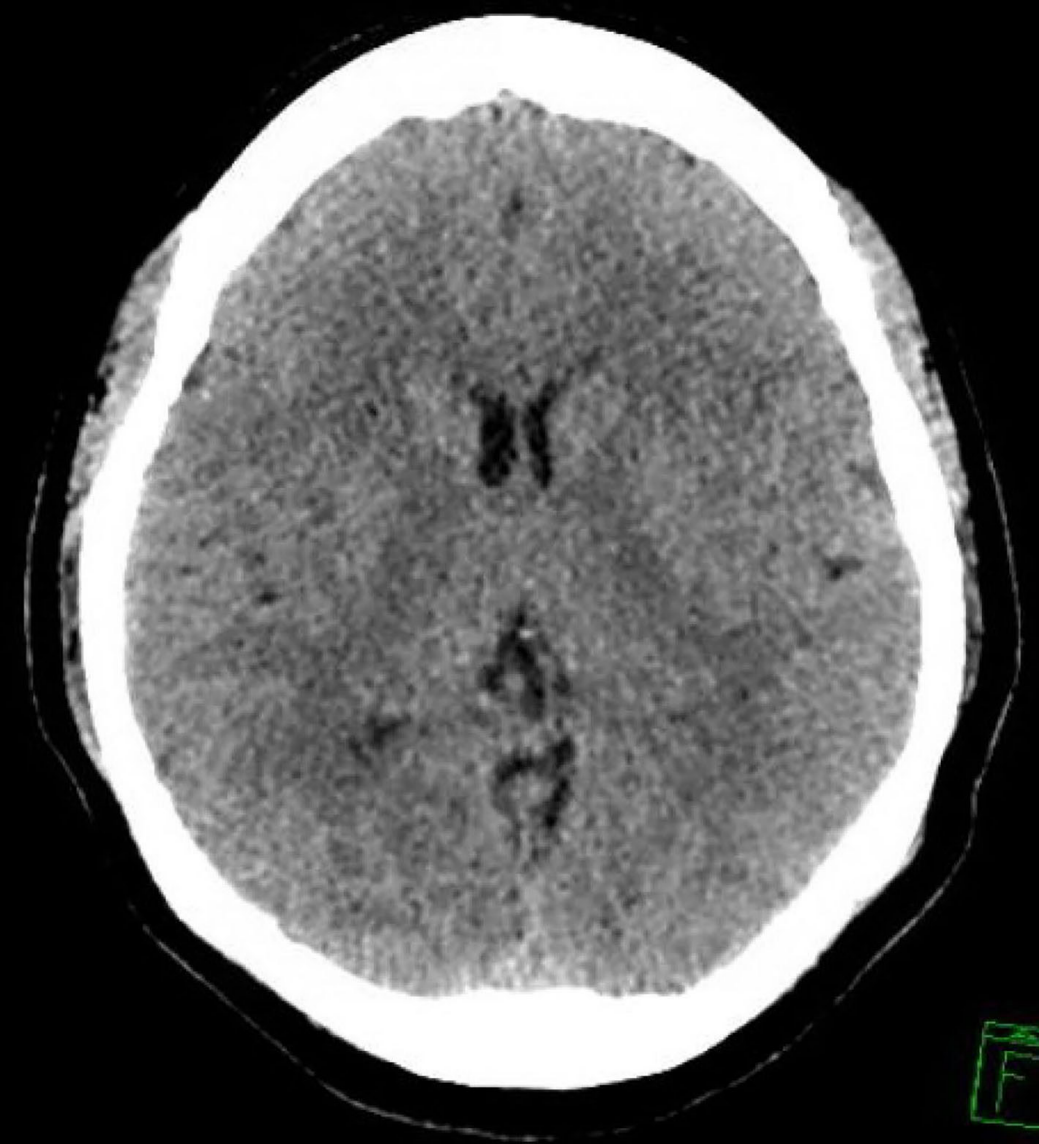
Computed tomography axial image demonstrating mild cerebral edema.
